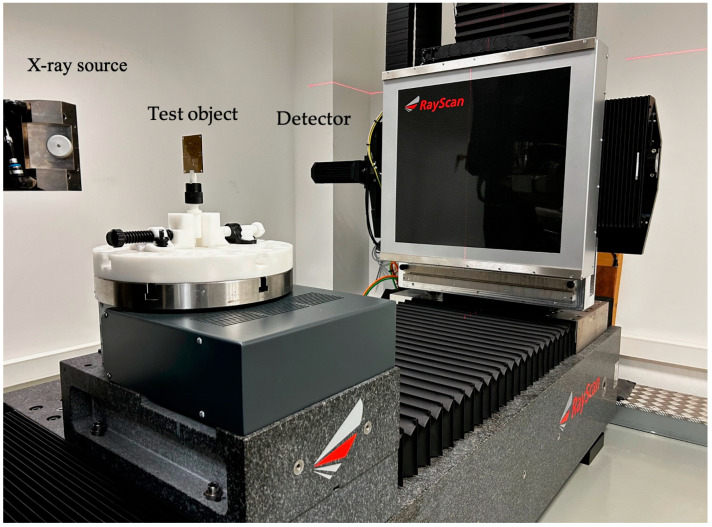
Photo of the experimental setup for radiographic testing.
